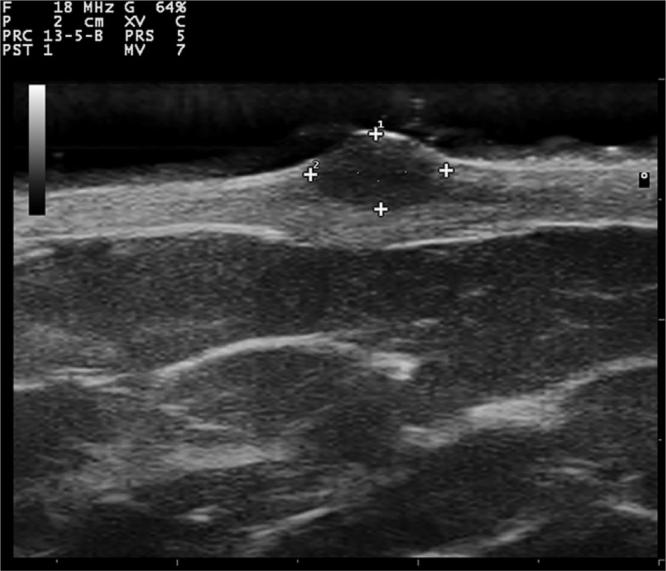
Transverse sonogram (18 MHz) of a superficial myxoma, showing a well-defined, round, homogeneous, and hypoechoic lesion located in the superficial dermis with elevation of the epidermis.
